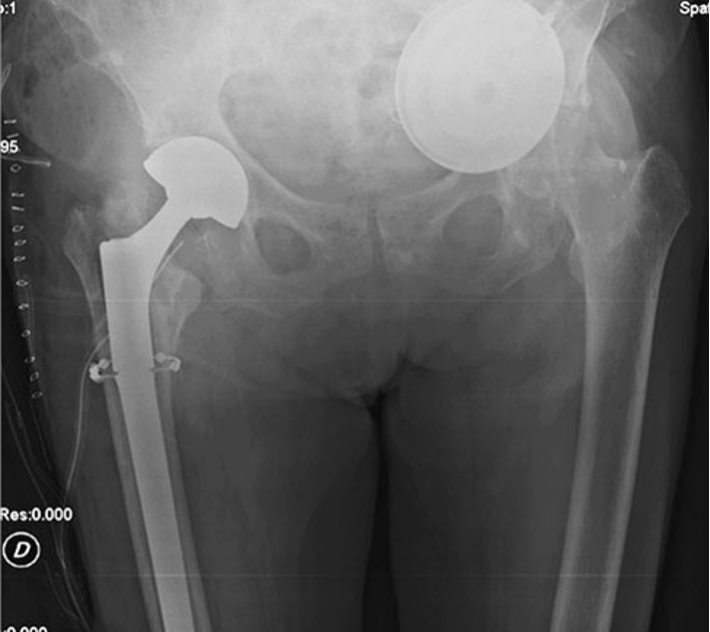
Postoperative radiograph after total hip arthroplasty
